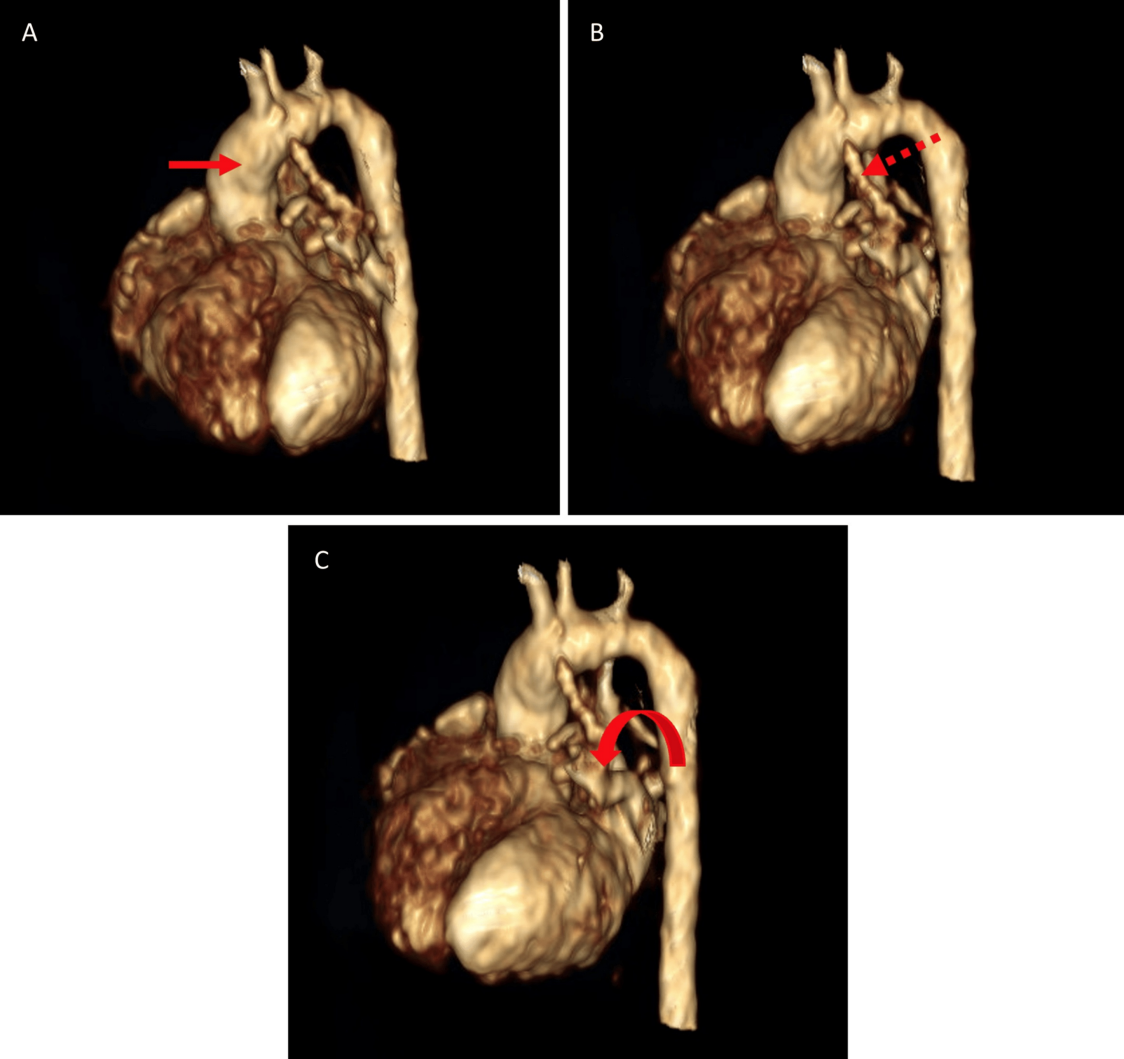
3D reconstruction of cardiac CT angiography. This image shows the ascending aorta (red arrow in A), persistent ductus arteriosus (dashed arrow in B), and hypoplasia of the pulmonary trunk and arteries (curved arrow in C).
